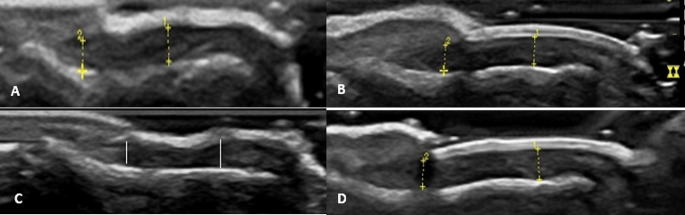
Ultrasonographic assessment of laser-treated and control nails of another patient at baseline and follow up
